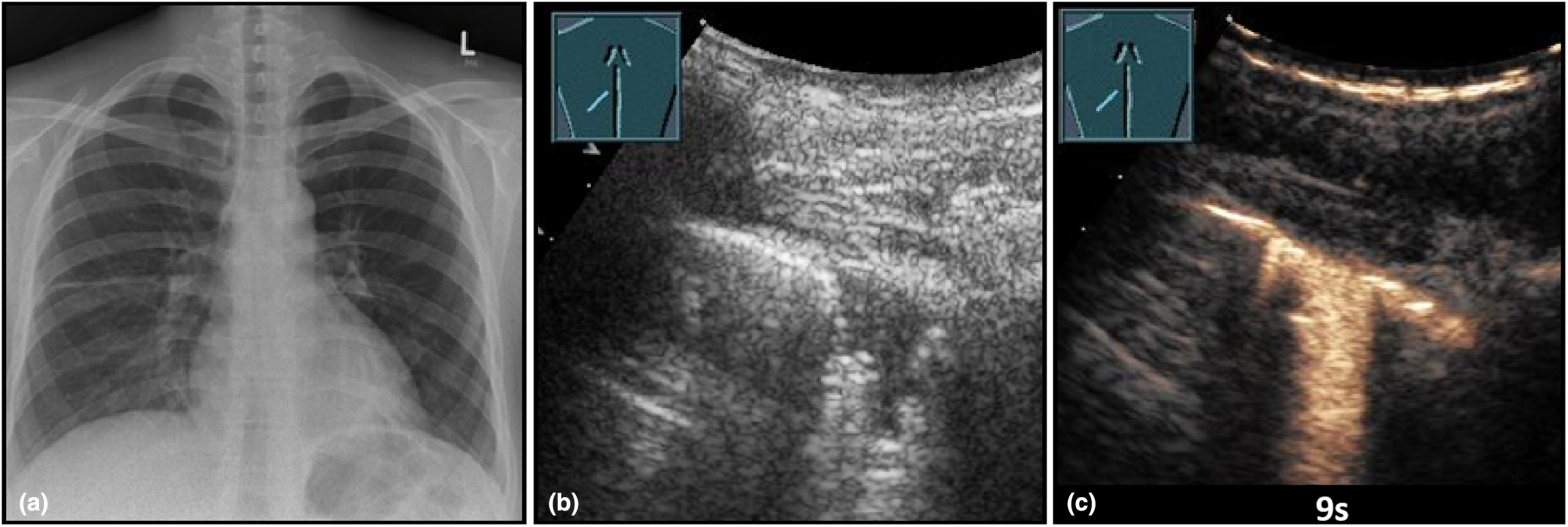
A 30‐year‐old female patient presented to the emergency department with respiration‐dependent chest pain and fever. (a) Visualisation of an unremarkable chest X‐ray (courtesy of Prof. Dr. Andreas H. Mahnken, Department of Radiology, University Hospital Marburg); (b) B‐mode ultrasound shows a hypoechoic, wedge‐shaped, pleural consolidation; (c) on contrast‐enhanced ultrasound, the lesion shows a pulmonary arterial, homogeneous perfusion pattern of enhancement, consistent with community‐acquired pleurisy.
